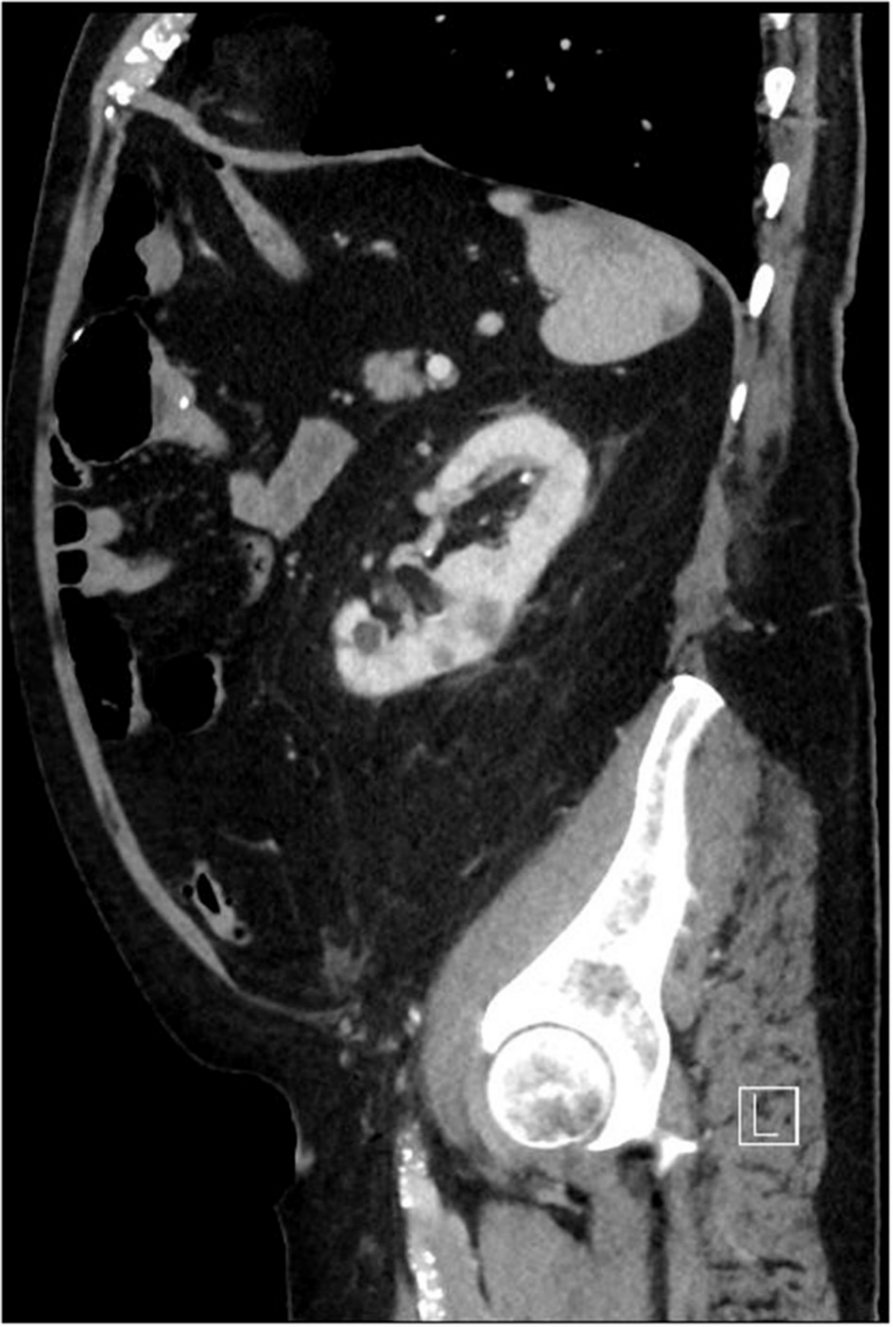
Computed tomography of the chest and abdomen showing septic embolism of the spleen and kidneys.
